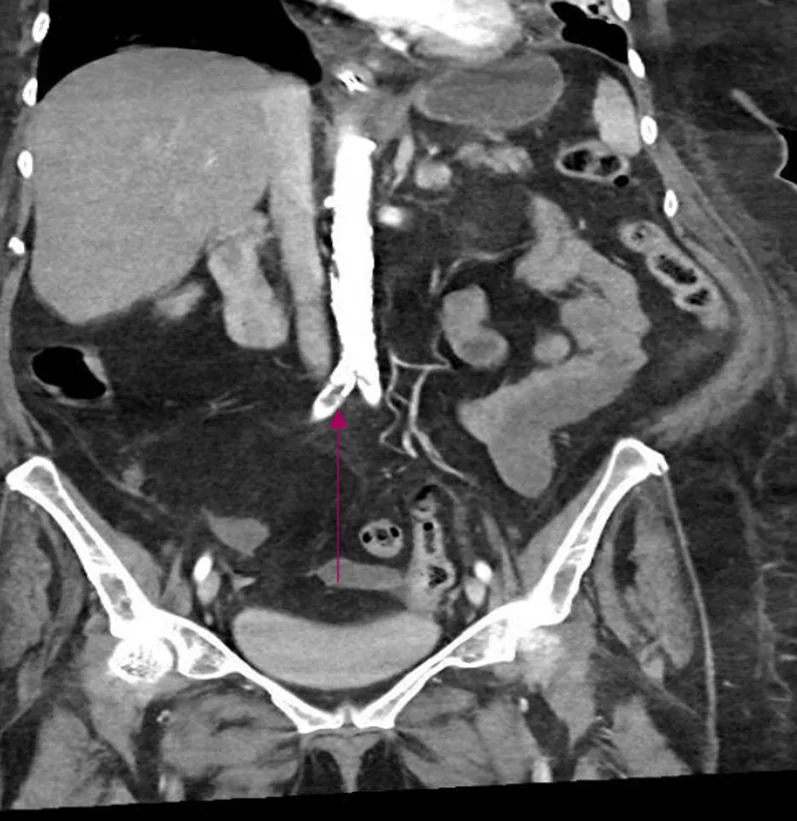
Acute Superior Mesenteric Artery Occlusion Coronal computed tomography angiography displaying the distal abdominal aorta splitting into the common iliac arteries. A large, prominent filling defect is tracking through the proximal right common iliac artery (arrow), causing subtotal luminal obstruction and a critical drop in downstream peripheral perfusion.
